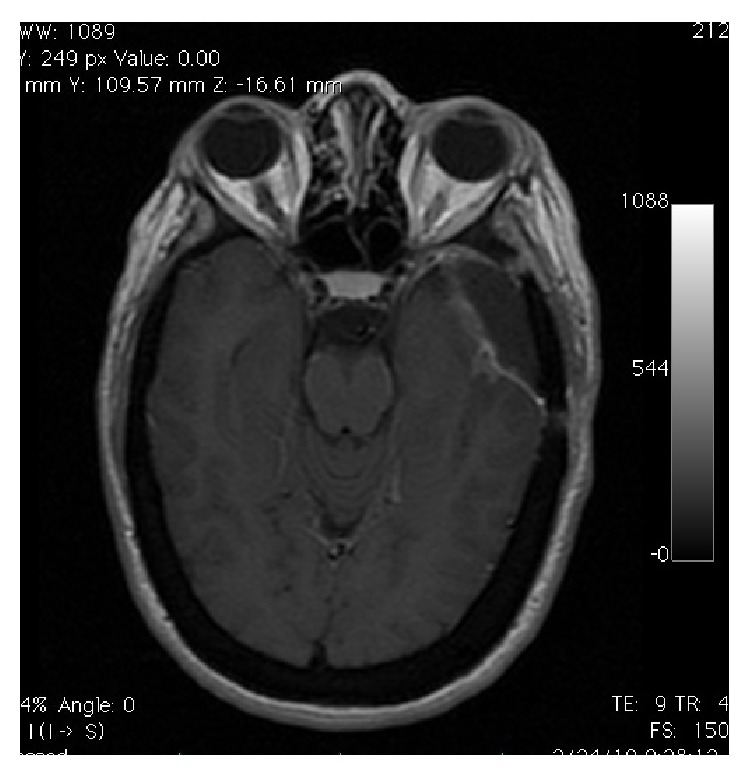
Postoperative (24 hr) follow-up T1-WI axial MRI demonstrates complete resection of tumor from the middle region.
